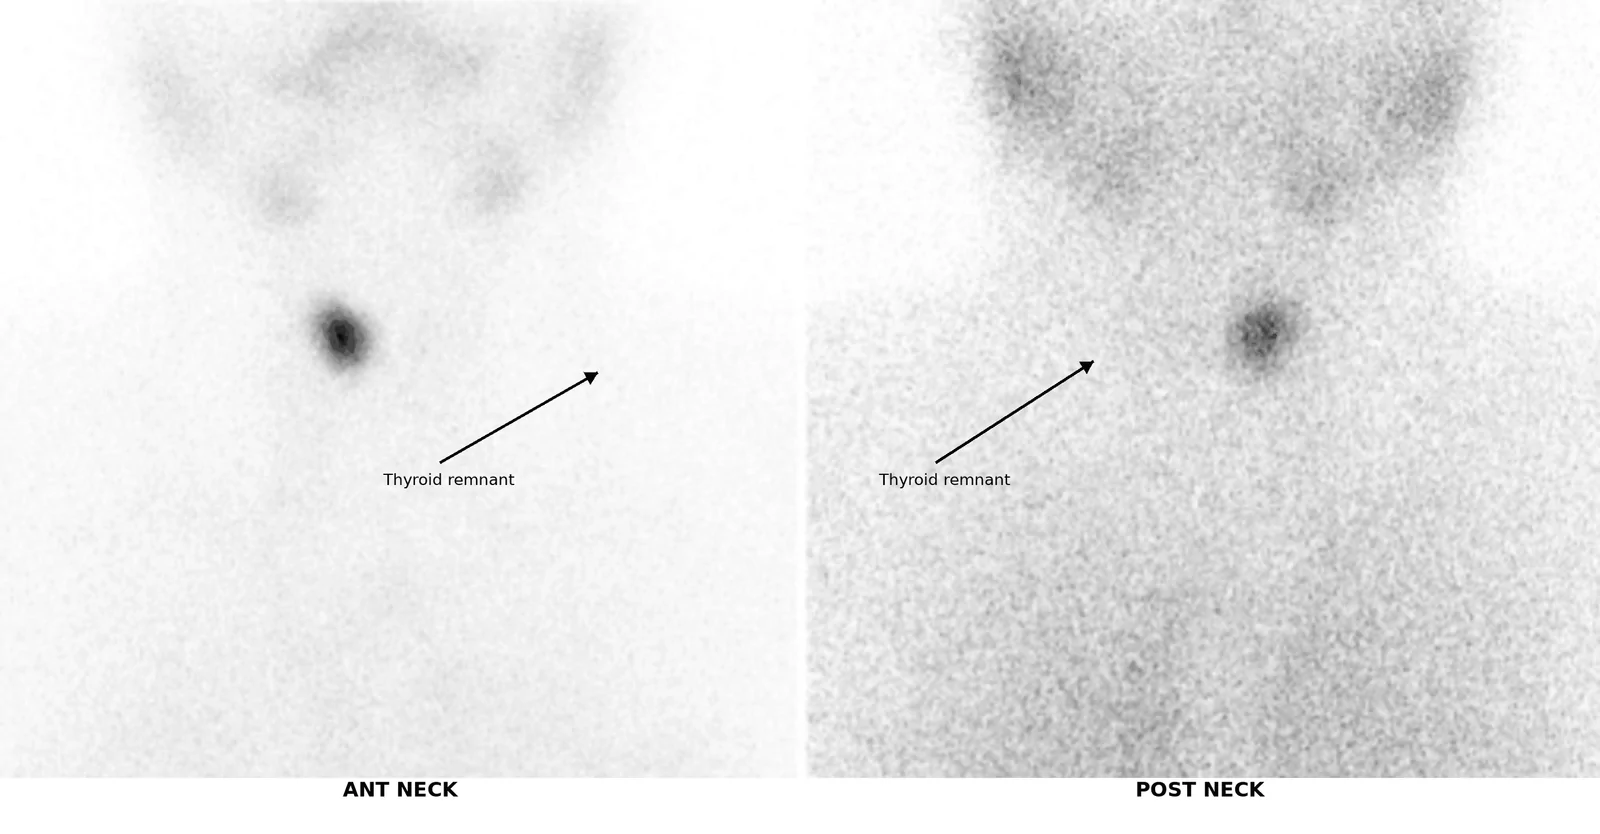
Thyroid scintigraphy demonstrating focal remnant thyroid tissue in the right thyroid lobe Anterior (ANT NECK) and posterior (POST NECK) thyroid scintigraphy images obtained after the administration of 5 mCi of 99mTc-pertechnetate, following left thyroid loboisthmusectomy. A focal area of increased uptake is identified in the topography corresponding to the superior pole of the right thyroid lobe, consistent with residual thyroid tissue of limited extension. This finding confirmed incomplete thyroidectomy and prompted completion right loboisthmusectomy to optimize conditions for subsequent radioiodine (^131^I) ablation.
